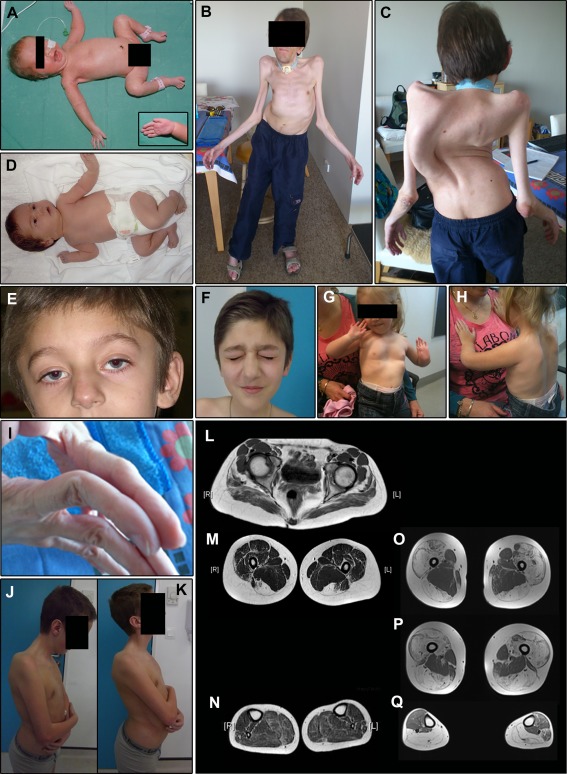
Examples of common and clinically significant features. Lower limb magnetic resonance imaging (MRI) is also shown. (A) Female Family 25 proband. The pregnancy for this infant was complicated by reduced fetal movements and prenatal ultrasound detection of limb contractures. The image shows typical “frog leg” positioning due to marked congenital hypotonia and weakness. Note also the nasogastric feeding tube and reduced palmar creases *(inset)*. This infant also had mild pulmonary stenosis and needed intubation and ventilation for a short period following delivery. (B, C) The older of the 2 affected brothers (Sibling 1) from Family 1 at age 34 years. Both brothers had congenital scoliosis, which progressed during childhood. Spinal surgery was not possible in the pictured brother due to concurrent development of severe respiratory insufficiency. His respiratory deficit was diagnosed at age 13 years following an out of hospital respiratory arrest, and he has since relied on nocturnal ventilation via a tracheostomy tube. This patient has slowly progressive mild to moderate limb weakness but remains ambulant despite significant axial involvement. He also has left ventricular cardiac dysfunction. In addition, these images demonstrate bilateral non‐congenital elbow contractures and marked generalized muscle hypotrophy. (D) Family 24 female proband who was born following a pregnancy complicated by reduced fetal movements and breech presentation. She was significantly hypotonic at birth and had congenital bilateral wrist contractures and fixed foot deformities. (E, F) Ptosis and facial weakness in the younger sibling (Sibling 2) from Family 26 during early childhood (E) and at age 12 years (F). (G, H) The same child as shown in (A) at age 2 years with a pectus excavatum chest wall deformity (G) and scapular winging (H). (I) Distal joint hypermobility in Sibling 1 from Family 1. (J, K) Reduced range of neck movement in Sibling 1 from Family 26. (L–N) Lower limb MRI result for Sibling 1 from Family 26 at age 15 years. This shows fatty infiltration of the gluteal muscles (L), severe fatty replacement of both visible hamstring muscles (complete fatty replacement of semitendinosus and incomplete but marked replacement of biceps femoris; M), relative sparing of the adductor compartment, and mild involvement of the calf and peroneal muscles (N). (O–Q) Lower limb MRI result for the Family 31 proband at age 29 years. This is one of the 4 segregation‐inconclusive cases described in this paper, but not included within the clinical analysis cohort. The features in this individual's lower limb MRI may represent the more severe end of the spectrum of muscle involvement associated with this disorder. The images show severe fatty replacement of the quadriceps and hamstrings, sparing of the adductors (also seen in the first MRI case), and severe replacement of both calves and the right peroneus, with mild fatty marbling in the anterior and lateral compartment on the left. Consent was obtained from patients/parents/legal guardians for use of the clinical photographs shown in this figure, including the non‐obscured facial photographs shown in D–F.
